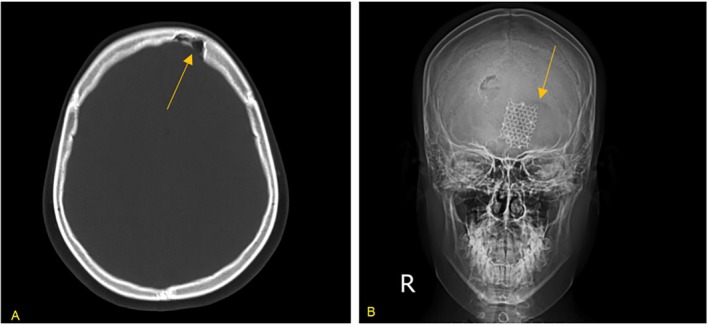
Skull imaging at the time of re‐presentation. (A) Axial CT scan showing changes at the margin of the left frontal bone resection site (arrow), suggesting local progression. (B) Frontal skull X‐ray demonstrating lucency surrounding the cranioplasty mesh (arrow), consistent with recurrence at the surgical margin.
